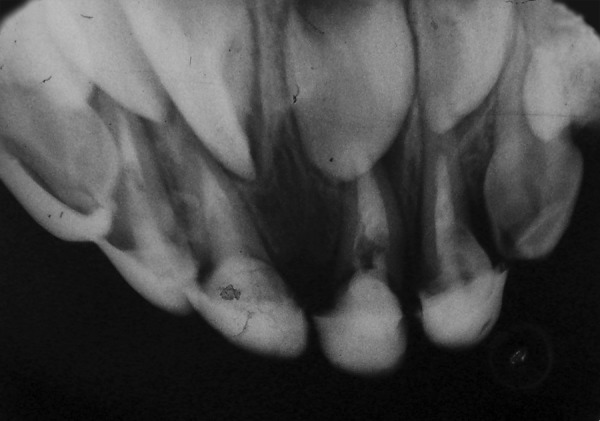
Radiograph after cementation of post
